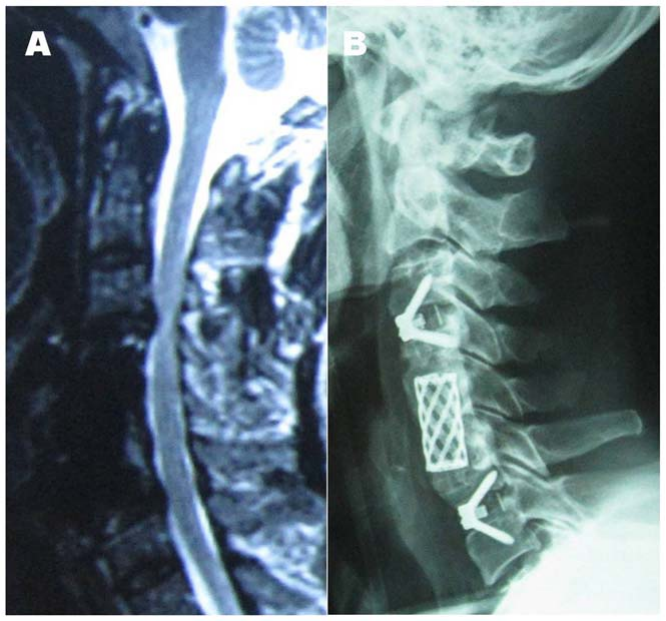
A 58-year-old male who received a reoperation for symptomatic adjacent segment disease. 1A. Postoperative MRI at the 6-year follow-up showed degeneration at upper and lower discs adjacent to the fused segments. 1B. Postoperative lateral X-ray on the second day after the second operation which performed by using Zero-P System (Synthes).
